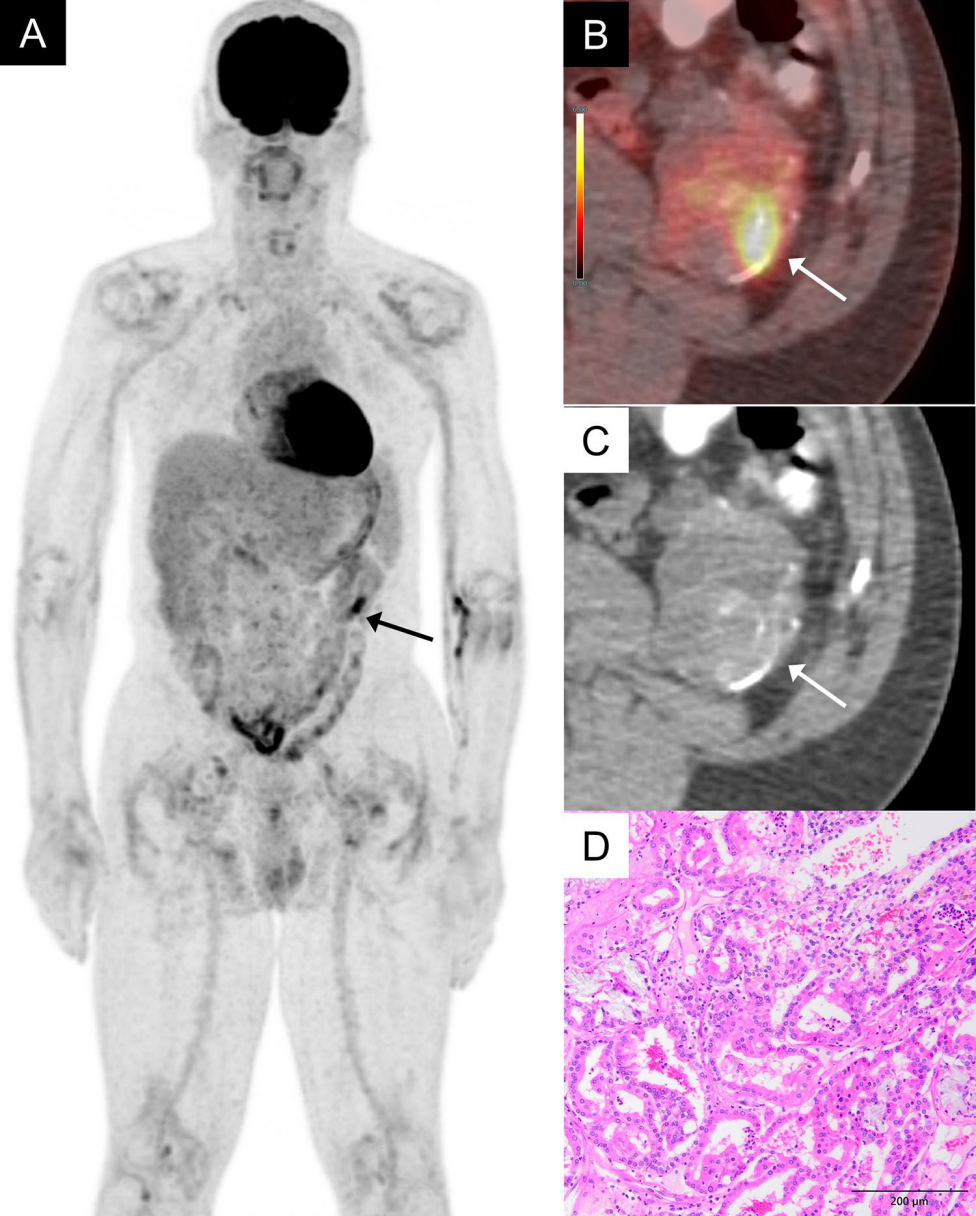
A 53-year-old man with an acquired cystic disease-associated renal cell carcinoma of the left kidney. A In the Maximum Intensity Projection (MIP) image of whole body, no FDG excretion into the renal urinary tract is observed, while localized accumulation (arrow) is noted in the left kidney. B Axial FDG-PET/CT image shows moderate FDG uptake in a mass of the left kidney (arrow), showing an SUVmax 6.9, while normal renal cortex shows an SUVmax 2.2. Notably, reduced kidney function leads to less FDG excretion through the kidney, which facilitates the detection of tumor. C It is difficult to identify the tumor on the CT scan. D The tumor shows tubular structures and calcium oxalate are observed in some areas. The tumor cells have distinct and eosinophilic nucleoli which are visible at low magnification (× 100)
